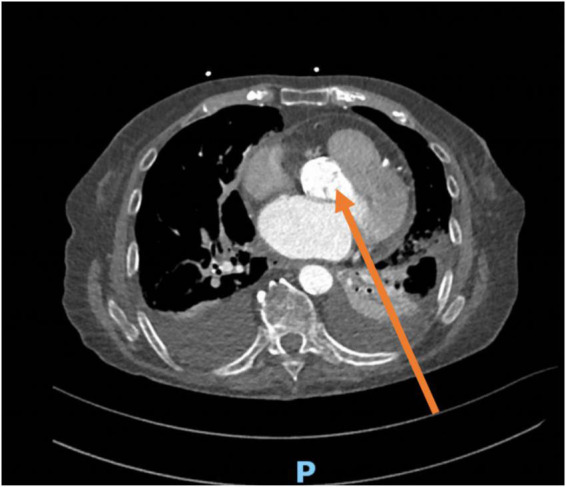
CT scan from the same patient showing the alignment of the Doppler beam in the posterior thoracic view toward the aortic valve (arrow). The transducer is positioned between posterior intercostal spaces, parallel to the ribs.
